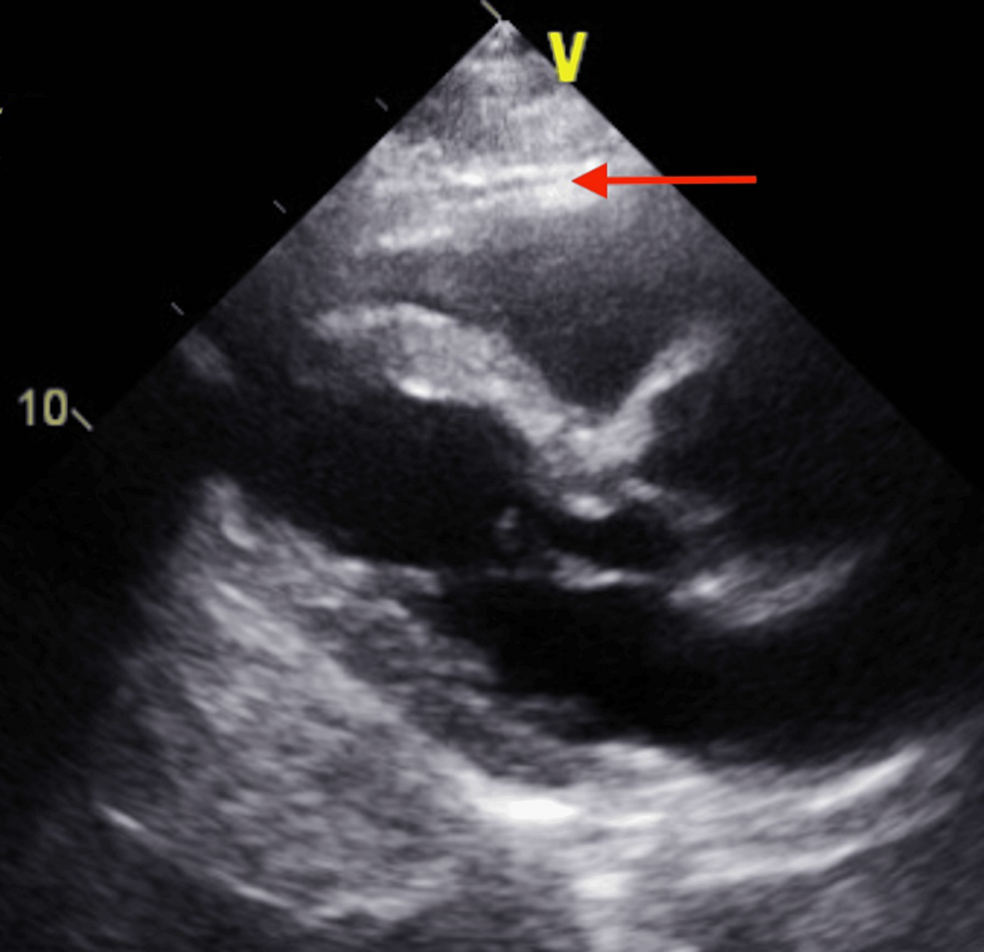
Transthoracic echocardiogram (TTE) on admission. TTE on admission long axis parasternal view shows minimal pericardial effusion (red arrow).
